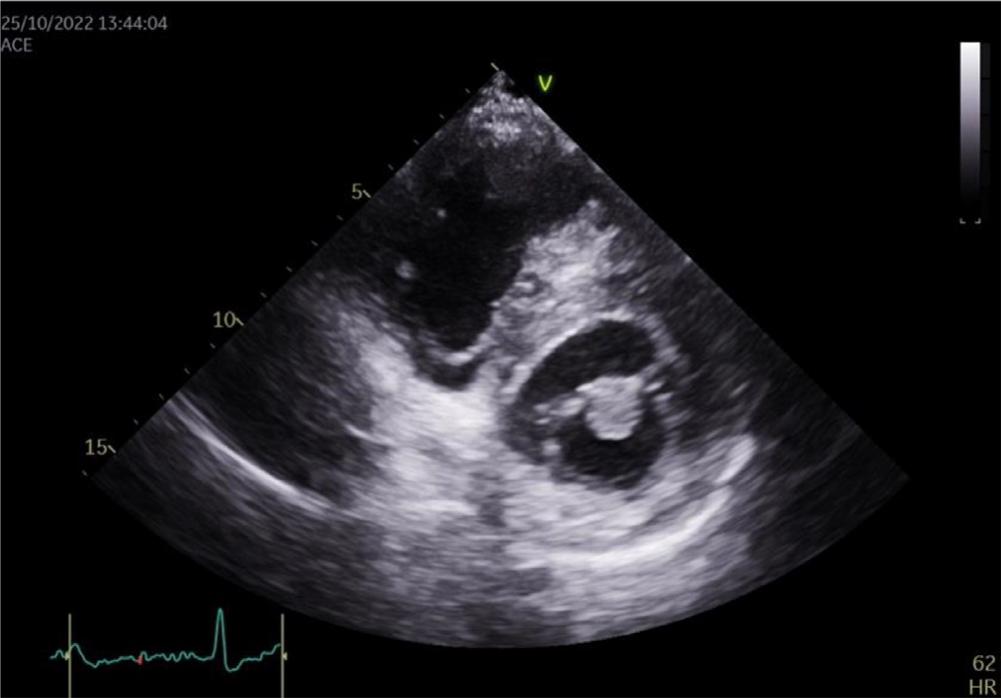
2D Transthoracic echocardiography image in the PSAX view showing a globular mass attached to the mitral valve.
